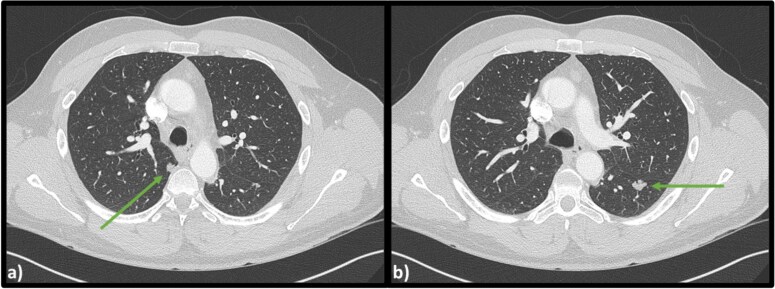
Axial CT scan of the chest indicating (a) lobulated lesion in apical segment of right lower lobe (9 × 10 × 10 mm) and (b) secondary lobulated lesion present in left lower lobe (15 × 11 × 14 mm).
